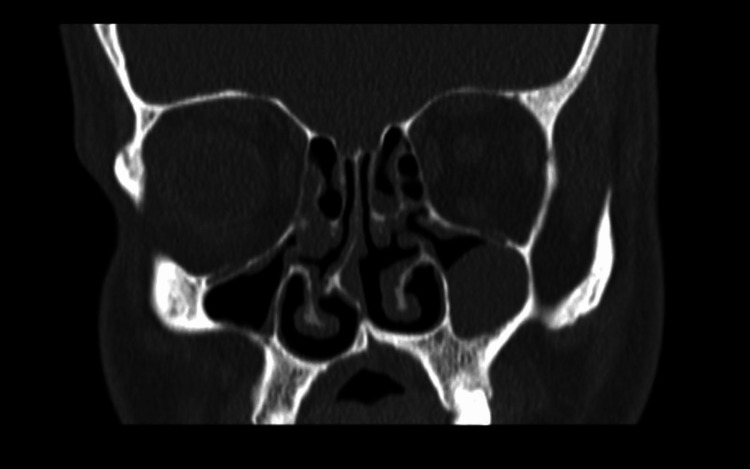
CT scan of the paranasal sinus, coronal view, showing a posterior-inferior defect in the bony septum, suggesting VA VA, vomer agenesis
